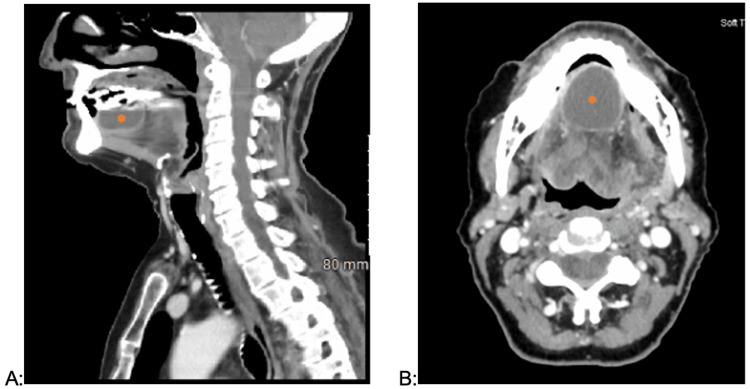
Contrast-enhanced CT imaging of the neck. Contrast-enhanced CT imaging of the neck demonstrated 2.9x3.0x1.5cm peripherally-enhancing fluid collection within the anterior tongue, suggestive of a recurrent lingual abscess. A) Sagittal view, B) Axial View. Asterisk labels lingual abscess.
